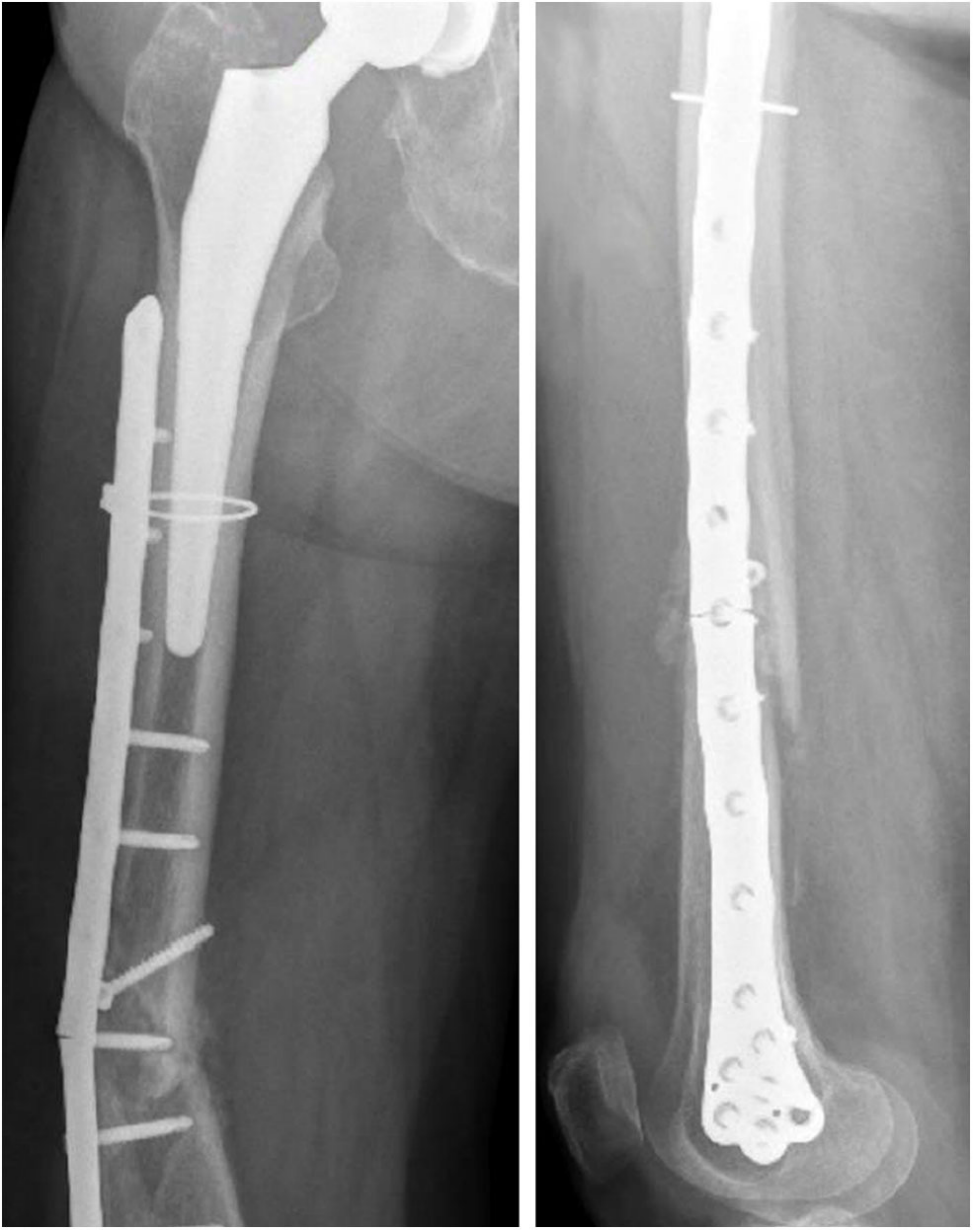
Anteroposterior (Left) and lateral (Right) x-ray images of the plate break.
